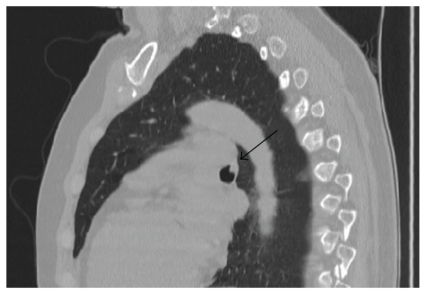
Three dimensional reconstructed CT image demonstrating an endobronchial soft tissue abnormality.
